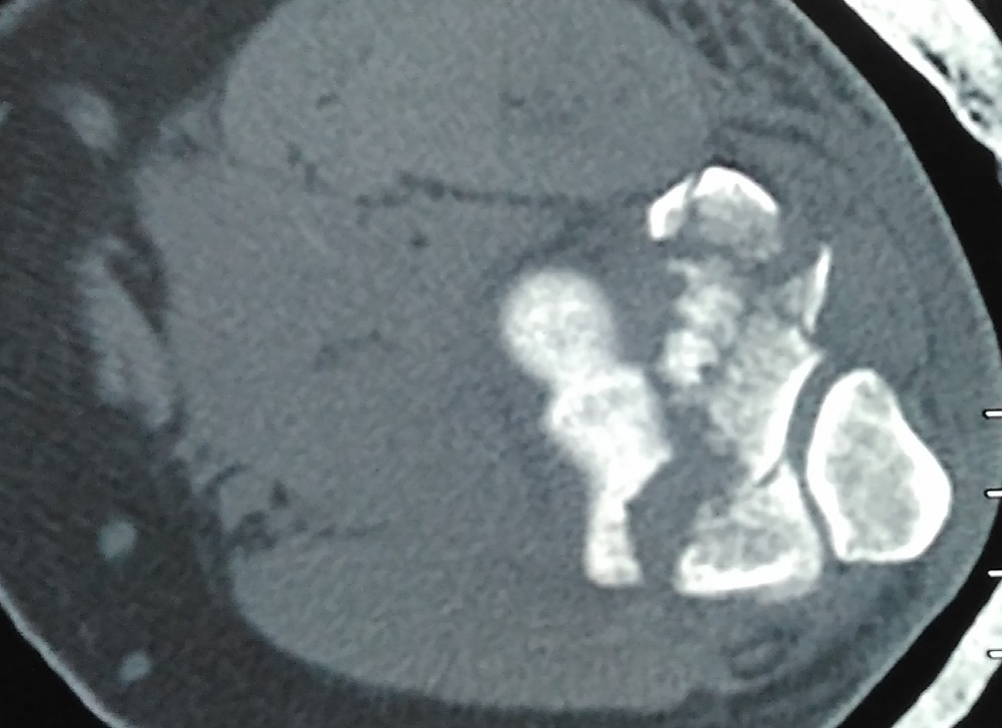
Axial Section CT scan showing a complex coronal plane fracture of distal humerus with posterior and lateral comminution.
